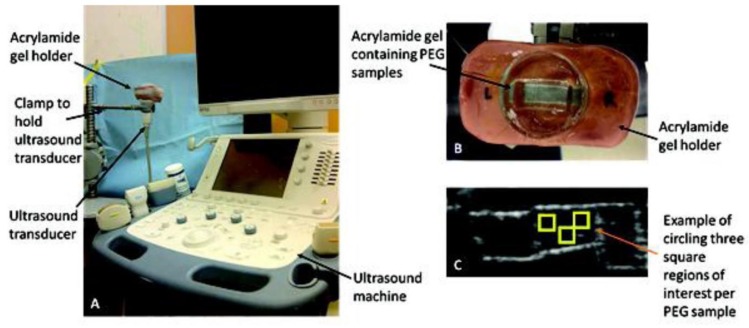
(A,B) Experimental setup of ultrasound used to image different areas of a scaffold made of PEG hydrogel. (C) Example of output image from the system. Reproduced with permission from Ref. [96].
